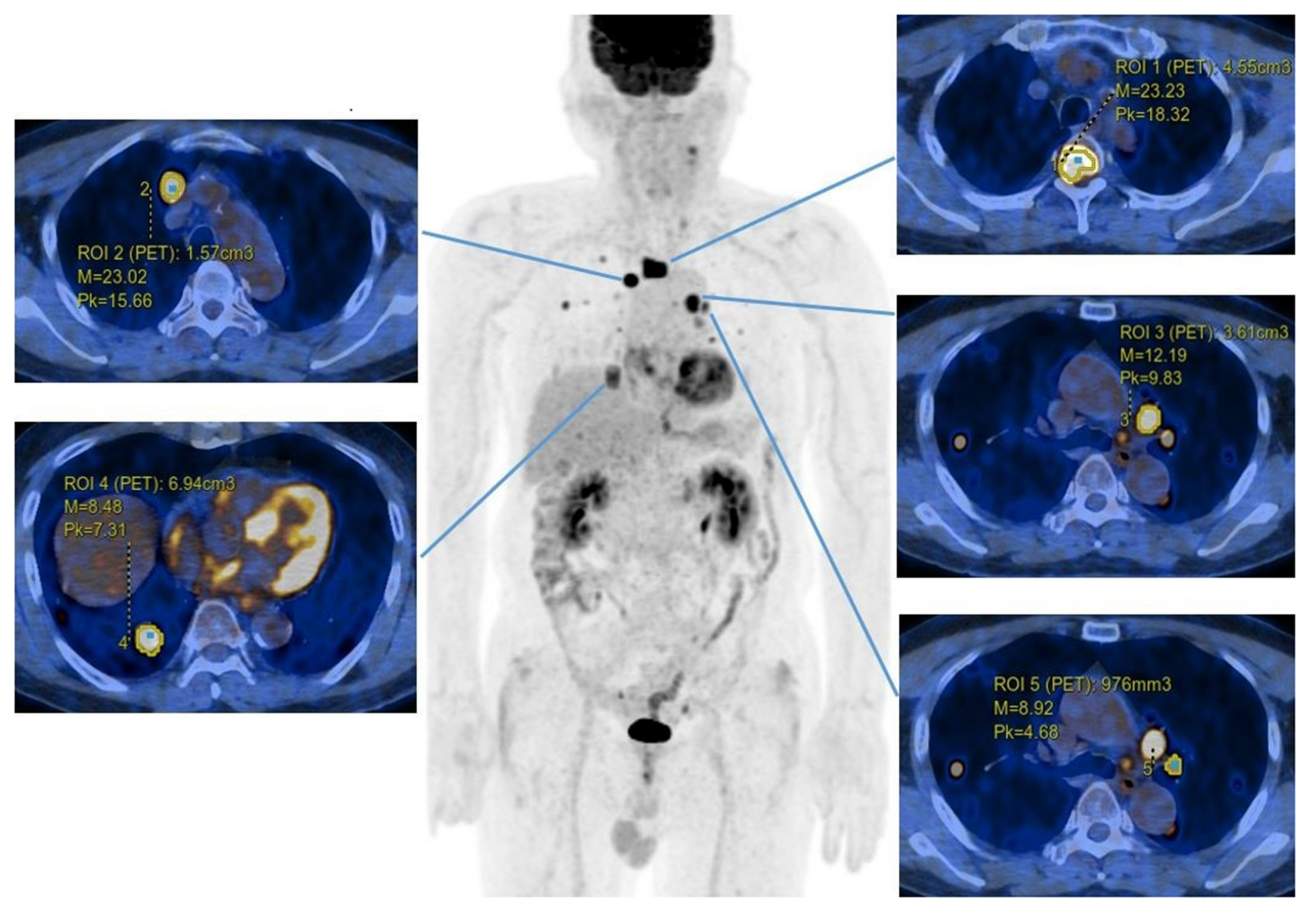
Representative image of lesion measurement. PET parameters were measured in a 71-year-old male patient with multiple metastatic lesions in both lungs, mediastinal lymph nodes, and a bone. The margins within the volume of interests were automatically created according to the 40% threshold of SUV_max_. MTV, SUV_max_, and SUV_peak_ are displayed in the axial fusion images.
